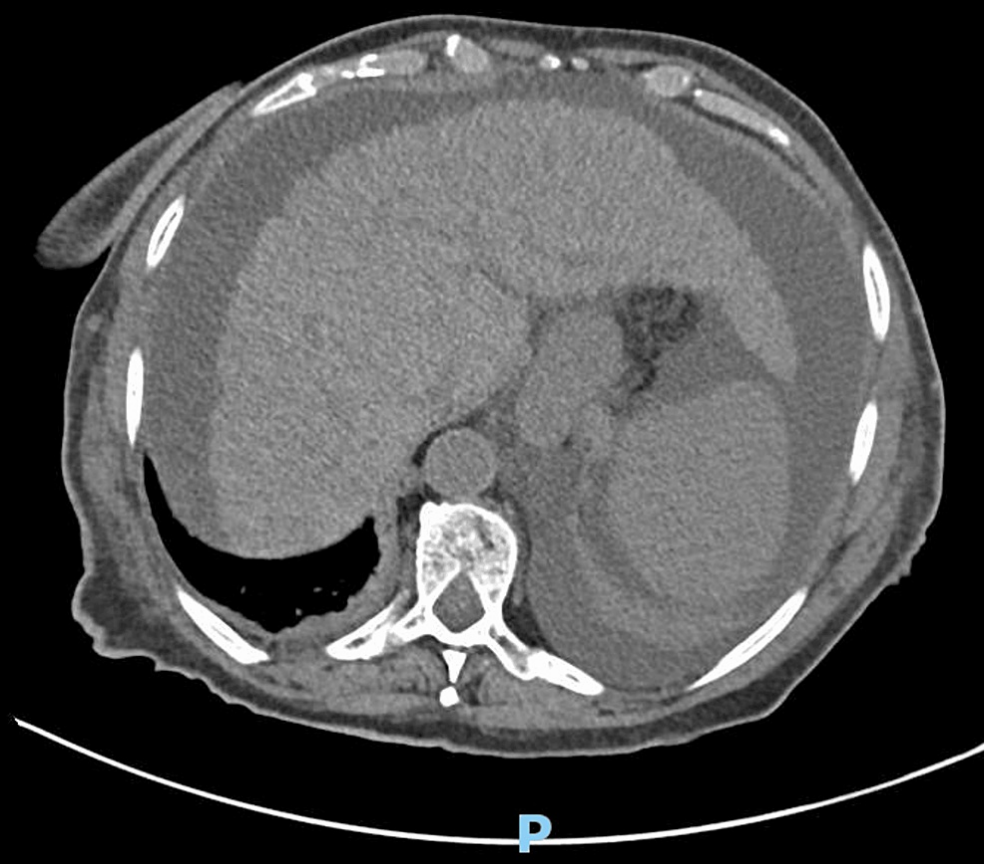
Abdominal computed tomography (axial section) Liver characteristics of chronic liver disease and evidence of small perihepatic ascites.
